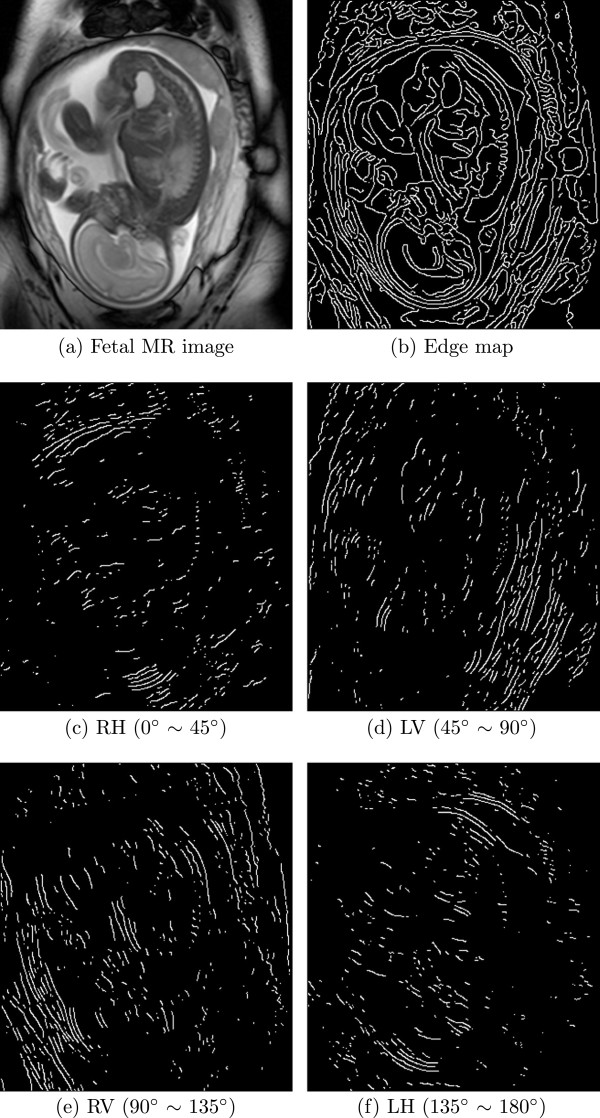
Edge map and orientations of edge points. (a) is the fetal spine MR image. Its resolution is 512 ×448. (b) is edge map extracted by Canny detector. *RH *(c), *LV *(d), *RV *(e) and *LH *(f) are shown with binary value.
